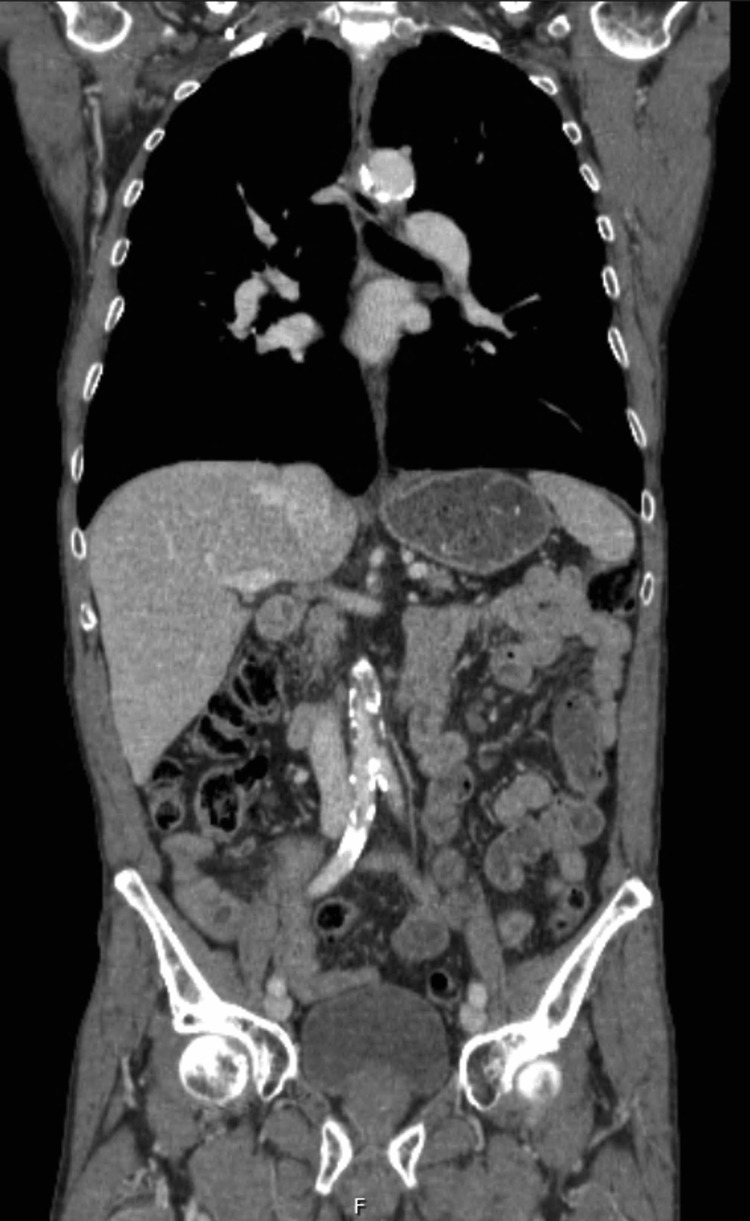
Abdominal and pelvic CT scan at postoperative follow-up shows resolution of the intestinal obstruction, with a normal caliber of the small intestine. CT: Computed tomography
